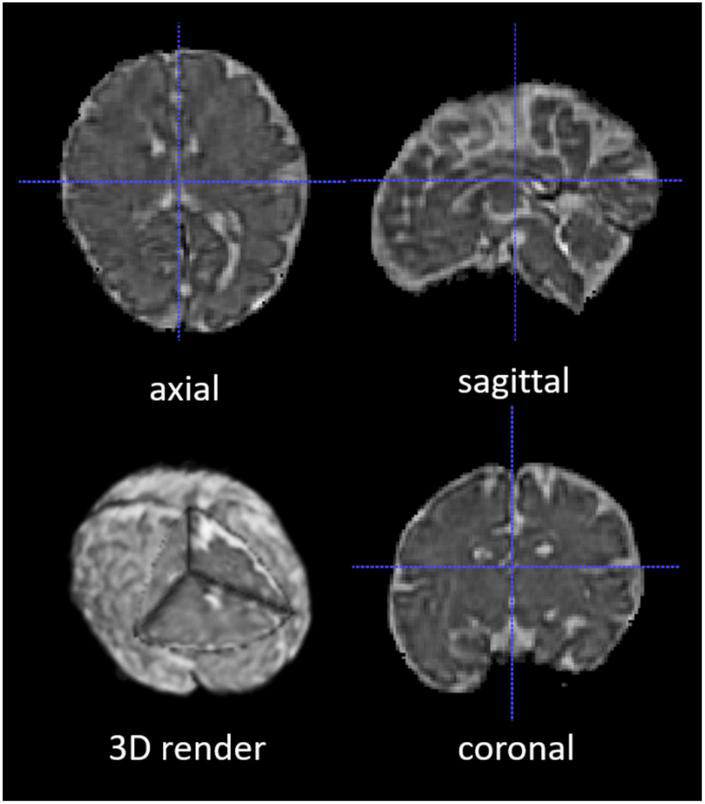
A segmented and volumetrically reconstructed fetal brain image using NiftyMIC. The original 2D slices of fetal MR images were automatically segmented and manually adjusted for fetal brain 2D masks. Then the 2D slices and 2D brain masks were reconstructed into 3D volumes and 3D masks with motion correction. This figure shows an example of the skull-stripped, orientation-adjusted 3D fetal brain volumes in axial, sagittal, coronal, and 3D-rendered views.
